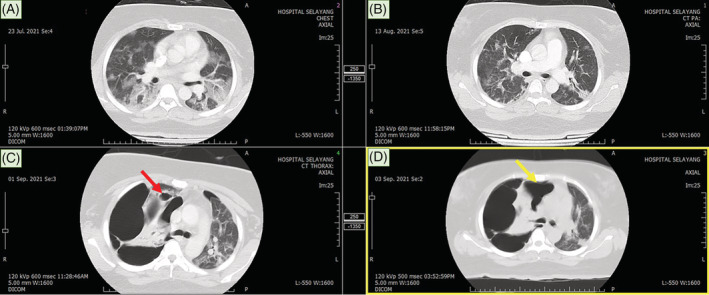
The subsequent CT thorax at day 4 of illness (image A) showed ground‐glass opacity and consolidation with predominant bilateral lower lobe distribution. Radiological improvement noted at day 25 post SARS‐CoV‐2 infection with residual ground‐glass opacity and subpleural curvilinear line (image B). Subsequent CT thorax at day 44 post SARS‐CoV‐2 infection (image C) revealed development of new cystic changes (red arrow) and multiseptated bullae with compressive effect and mediastinal shift. A ruptured cyst was identified leading to right loculated pneumothorax (yellow arrow).
